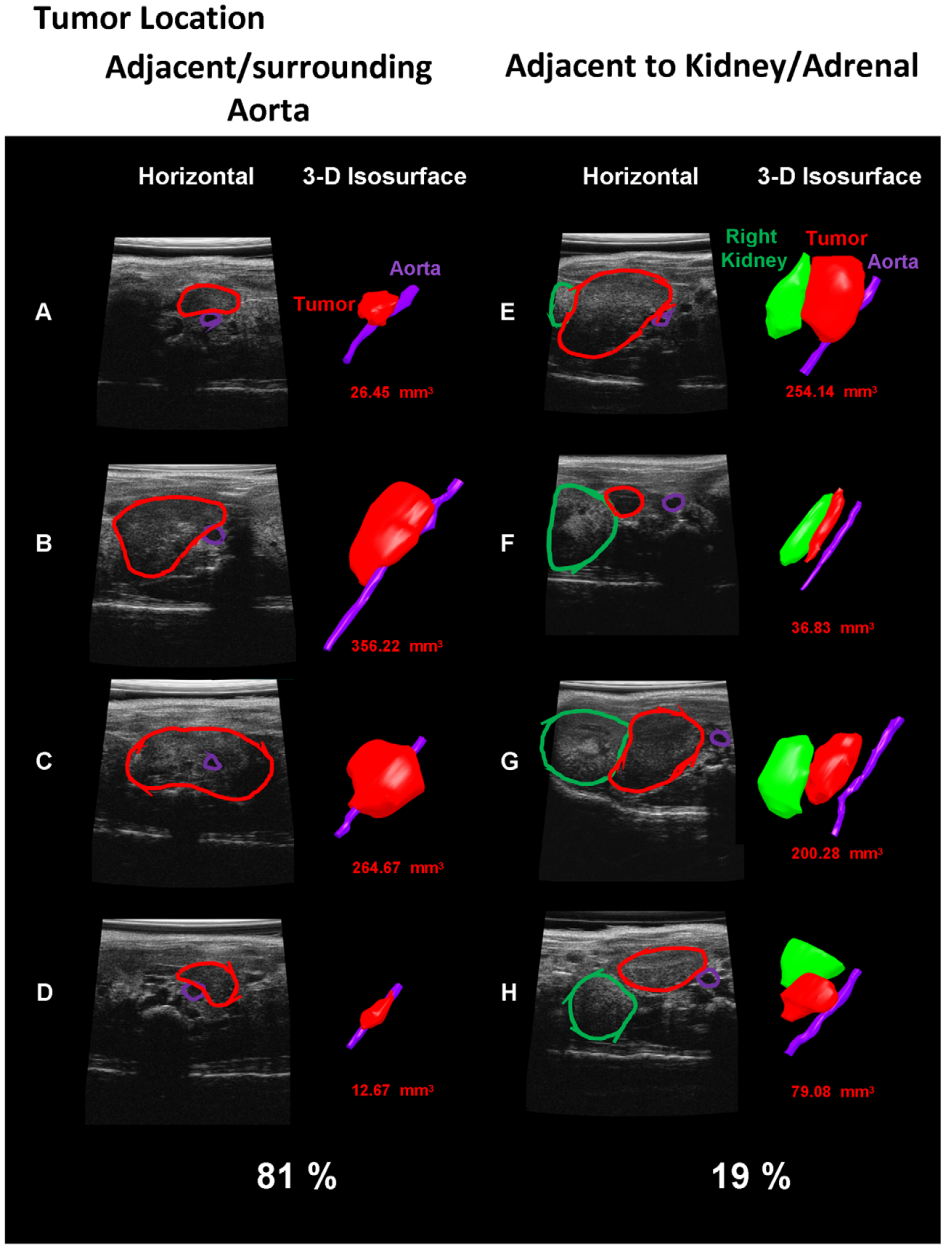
Location of TH-MYCN tumors as determined by ultrasound
